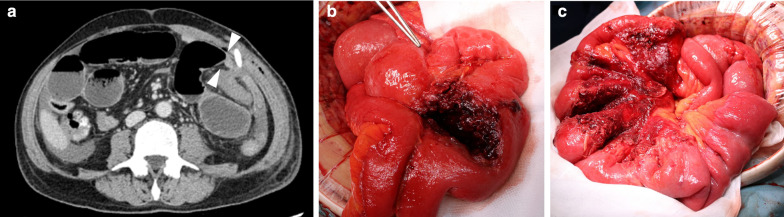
Computed tomography scan before re-operation and operative finding. Computed tomography revealed intra-abdominal hematoma and caliber changes in the small intestine (a arrowheads). The small intestine was obstructed by the intra-abdominal hematoma (b), and intestinal obstruction was released by removal of the hematoma (c)
